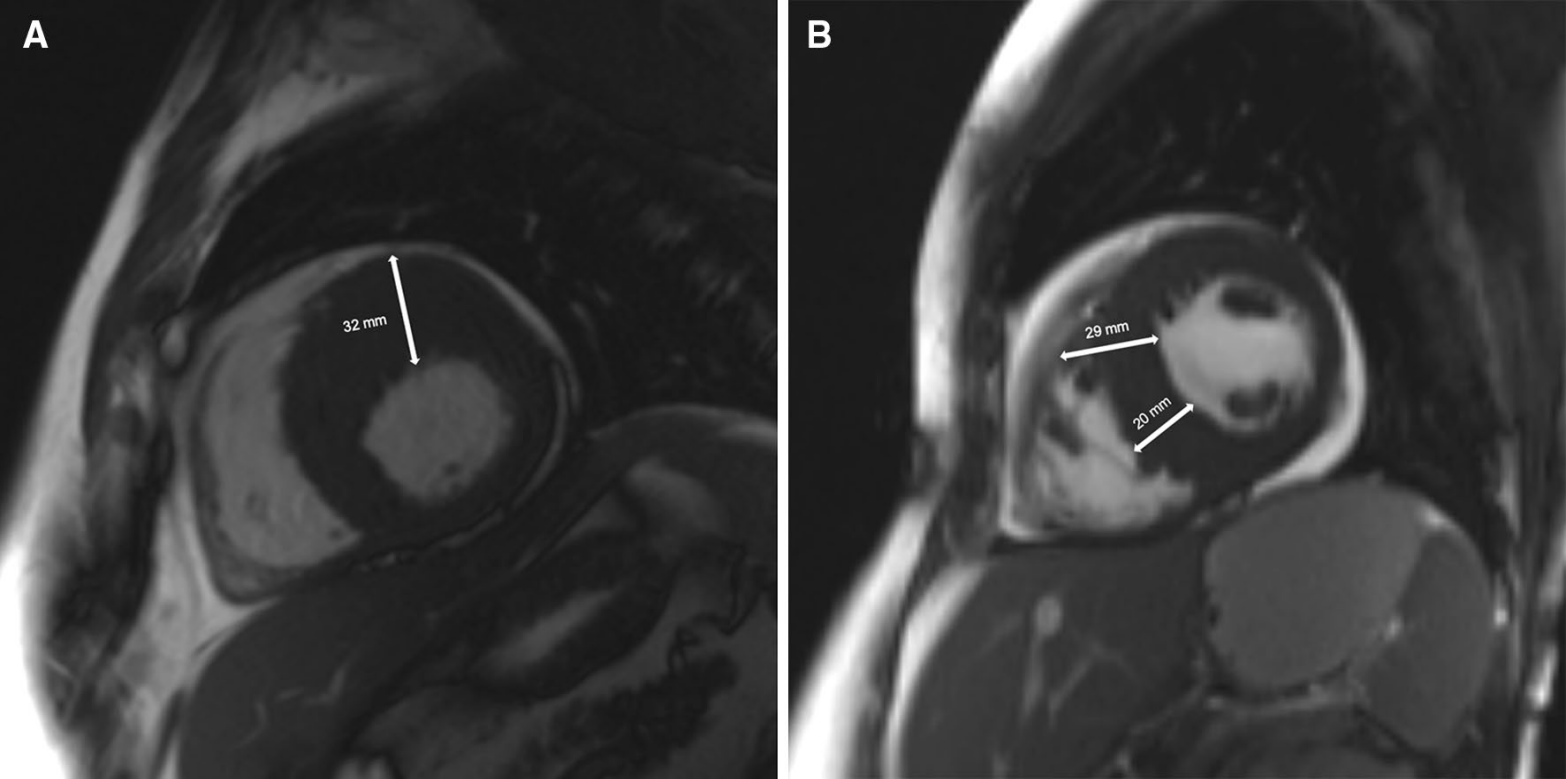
(A) Magnetic resonance image in short-axis slice demonstrating left ventricular hypertrophy with the maximal left ventricular wall thickness of 32 mm in basal anterior segment, which has been underestimated by 13 mm in transthoracic echocardiography. (B) Magnetic resonance image in short-axis slice demonstrating left ventricular hypertrophy with the maximal left ventricular wall thickness of 20 mm in mid infero-septal segment. The echocardiographic measurement of maximal left ventricular wall thickness (29 mm) was overestimated by inclusion of right ventricular trabeculae adjacent to the mid antero-septal segment.
